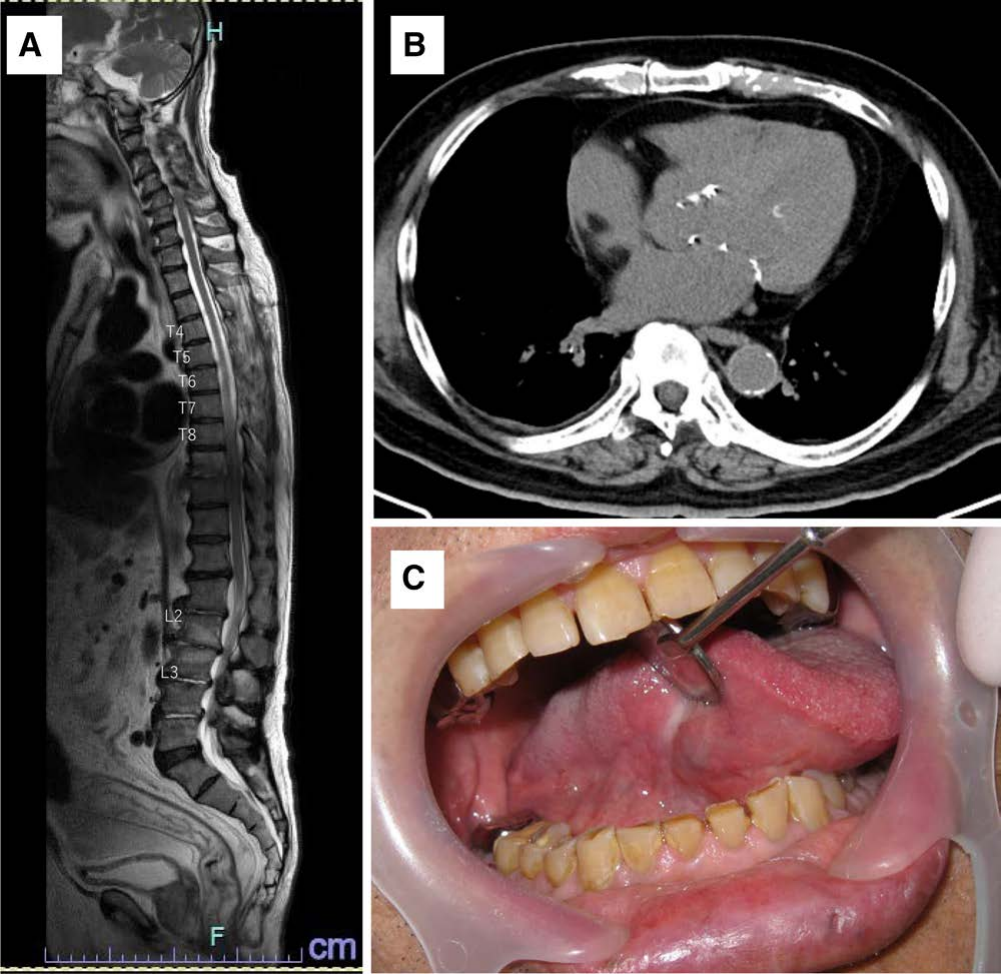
Imaging findings at the completion of treatment. (A) MRI (T2-weighted sequence) shows improvement in inflammatory findings throughout the spine, with no apparent abscesses. (B) No abscesses are observed. (C) No tongue cancer recurrence is observed. MRI = magnetic resonance imaging.
